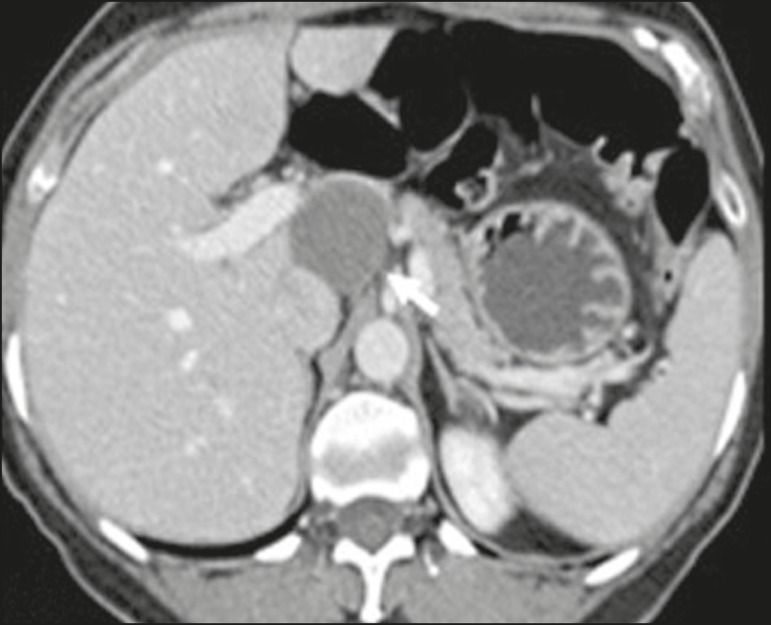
Ganglioneuroma in a 31-year-old female. CT scan showing a
